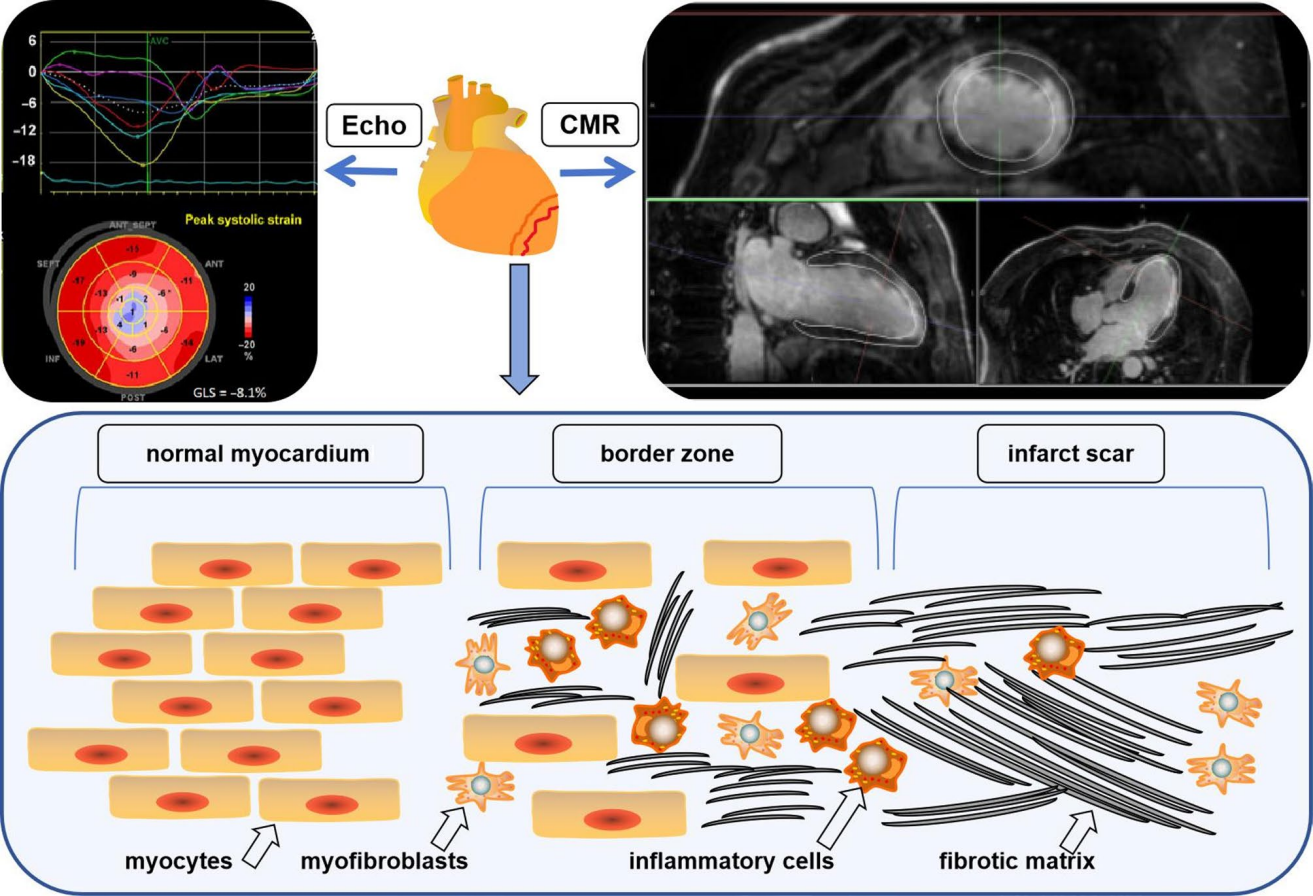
Imaging technologies applied to evaluate ventricular arrhythmia in ischemic cardiomyopathy patients. Echo, Echocardiography; CMR, cardiac magnetic resonance
